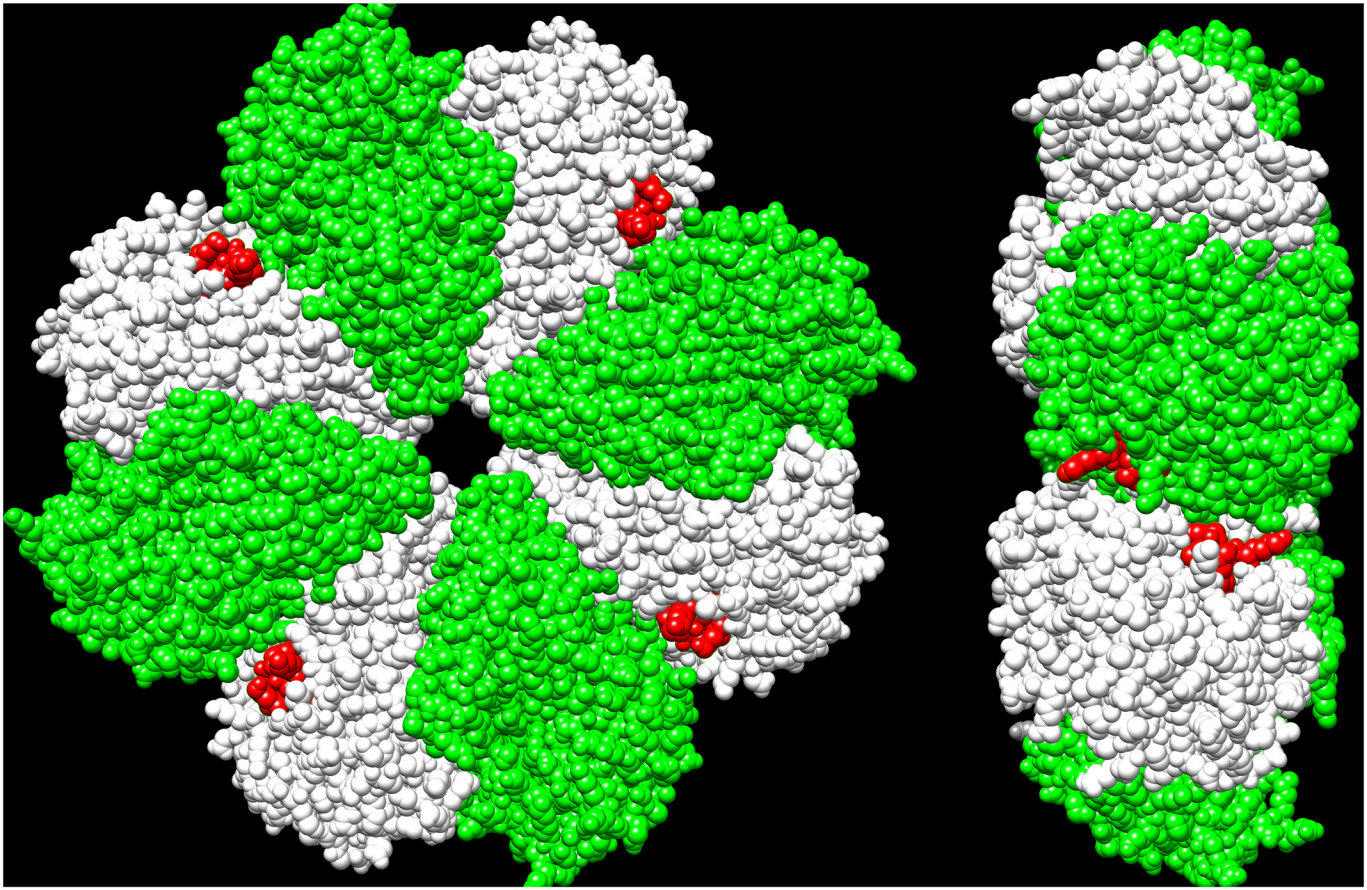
Str enolase (3ZLH.PDB) showing the alternating pattern of interfaces. The red represents residues 428–433 of the protein and indicates the position of the dimer-dimer interface; residues 434–435 do not appear in the X-ray structure and are not shown. The other interface is that of the monomer-monomer. Left side panel. Residues 428–433 appear on four subunits looking down on the donut and on the alternating subunits on the other side of the donut. Right side panel. Looking from the side of the donut clearly shows the nature of the dimer-dimer interface.
